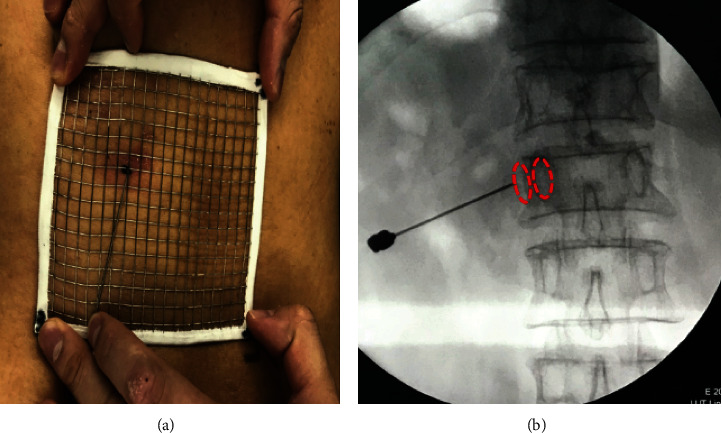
(a) Point A may be identified without the use of an X-ray fluoroscopic by using a body surface finder. (b) The junction of the central line of the transverse process and the lateral edge of the pedicle, which was horizontally translated one pedicle distance outwards, was Point B under X-ray fluoroscopic.
